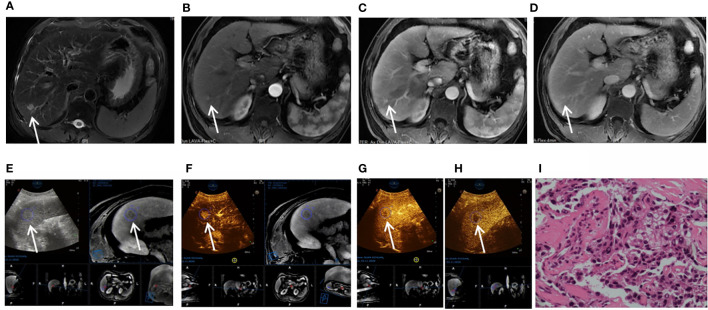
Images shown a 1.8 cm APHE nodule without washout in the venous and late phases in liver segment 6/7 in a 37-year-old man with chronic hepatitis B. (A) A high-signal nodule (arrow) was demonstrated in liver segment 6/7 on MR T2WI sequence. (B) In the arterial phase, hyperenhancement (arrow) was shown on CEMR. (C) Hyperenhancement (arrow) was observed in the venous phase. (D) Hyperenhancement (arrow) was observed in the late phase and was categorized as MRI LR-3. (E) After fusion imaging (ultrasound image on the left; real-time synchronous MR image on the right) navigation, the S6/7 nodule (arrow) was not displayed by conventional grayscale ultrasound on the background of liver cirrhosis. (F) FI-CEUS showed hyperenhancement (arrow) in the arterial phase compared with the surrounding liver parenchyma. (G, H) Mild washout (white arrow) was shown in the venous phase (G) and late phase (H) and was categorized as CEUS LR-5. (I) The patient underwent FI-CEUS-guided biopsy before microwave ablation, and the lesion was proven to be moderately differentiated HCC at pathologic analysis.
